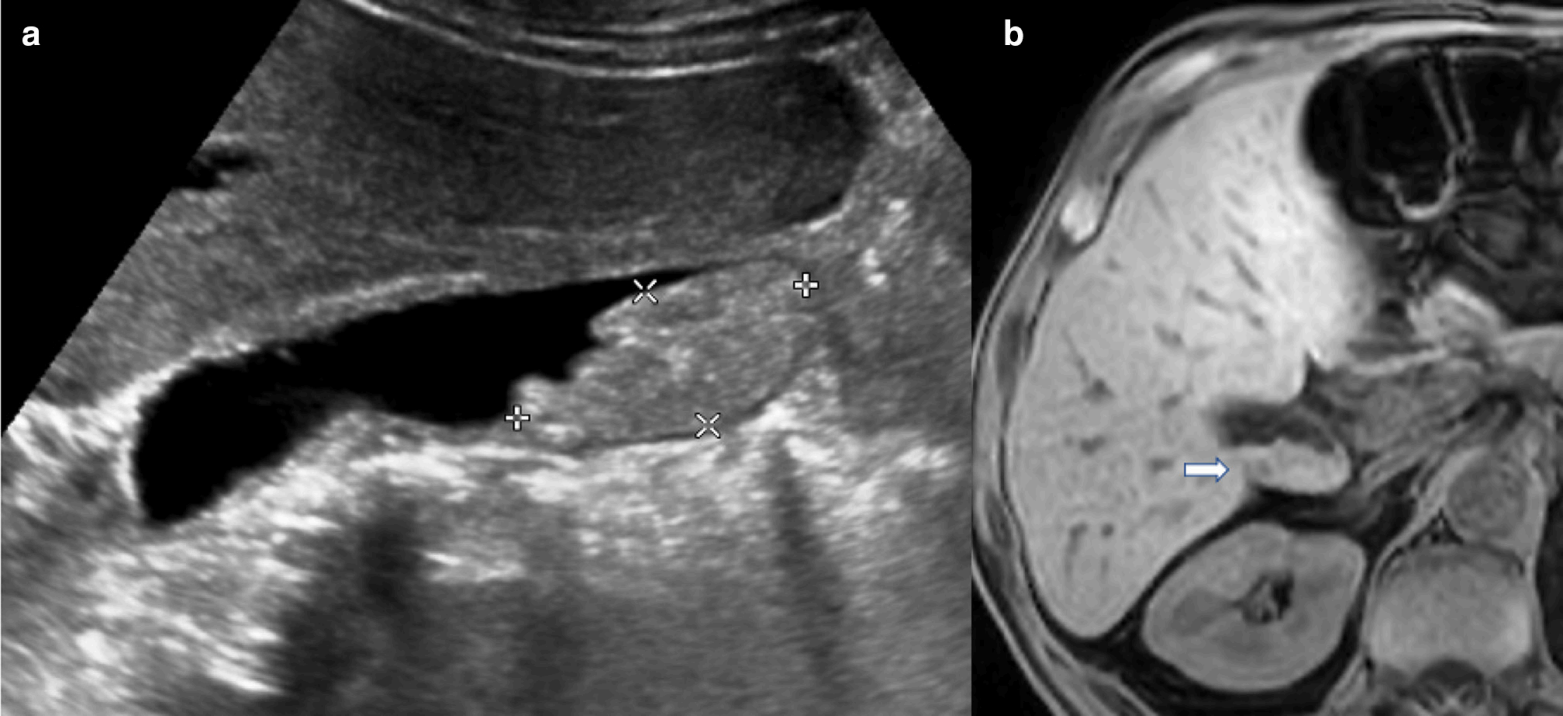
Sludge mimicking gallbladder pathology. (a) Greyscale ultrasound image in longitudinal section demonstrating a non-mobile gallbladder “polypoid mass”. (b) Unenhanced T1-VIBE image from subsequent MRI shows layering of high T1 signal within the gallbladder lumen, typical of the paramagnetic effect caused by metal ions within sludge, with no evidence of a soft tissue mass (white arrow). VIBE, volumetric interpolated breath-hold examination
